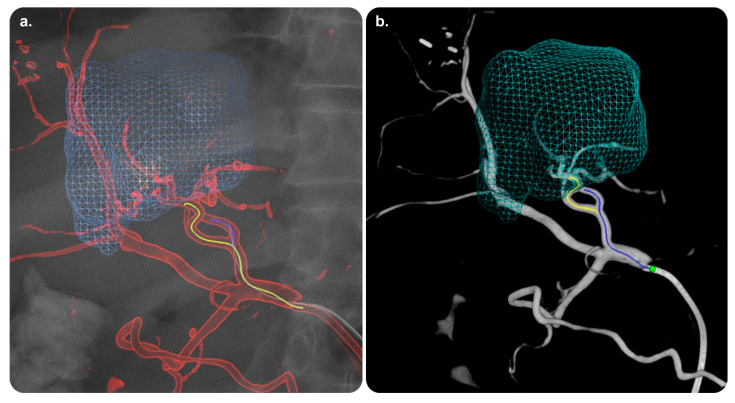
EmboGuide software and its role in the delivery of targeted therapy. (a) Feeding arteries superimposed on a live fluoroscopic image during selective catheterization. (b) 3D dynamic roadmap displayed on fluoroscopy after dual-phase CBCT enabling target volume segmentation, automatic feeding vessel detection, and selective microcatheterization of feeding vessels. Reprinted from “First human experience with directly image-able iodinated embolization microbeads” by Levy et al. [39] with written approval from the corresponding author and publisher (CardioVascular and Interventional Radiology; Copyright 2016).
